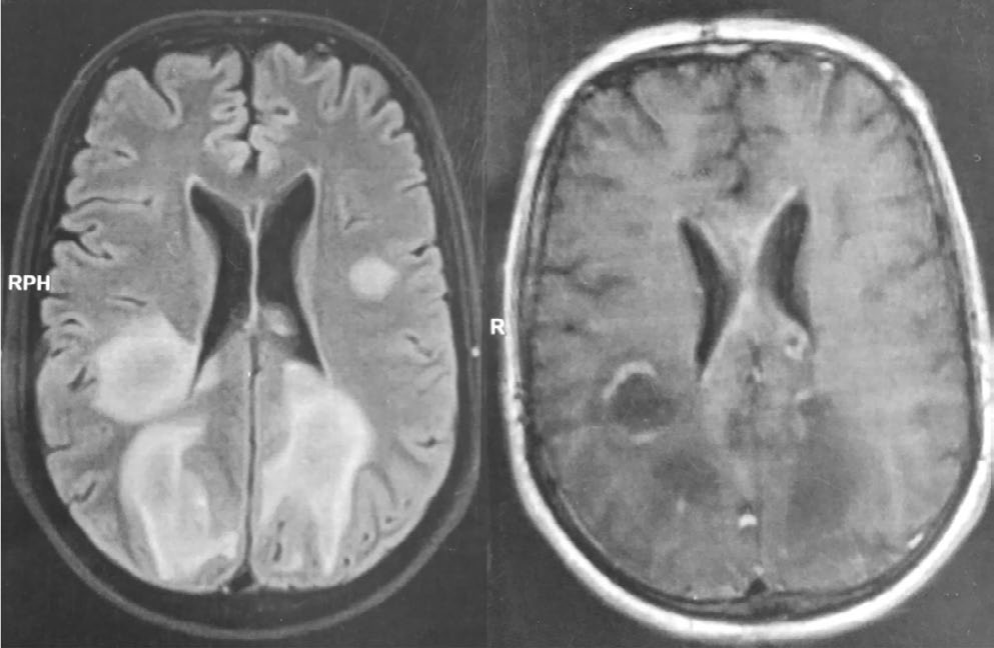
FIGURE 1 Brain magnetic resonance imaging (T1 GADO and Flair) showed multiple patchy and nodular lesions in the bilateral parieto‐occipital regions, with ring enhancement after gadolinium injection
